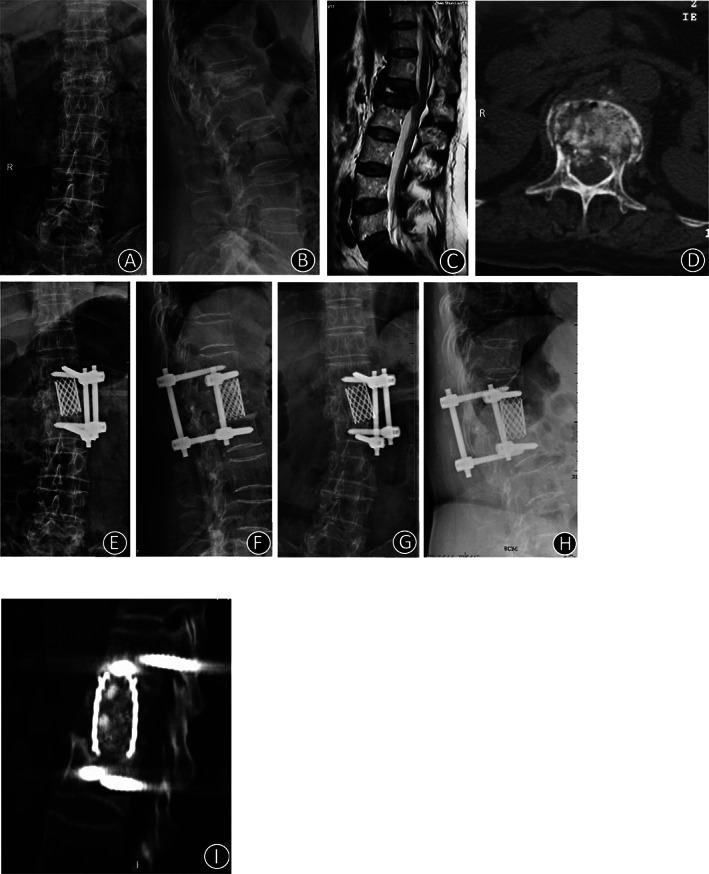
Clinical imaging from one representative patient (71‐year‐old woman) who complained of lumbar 1 compression fracture. (A, B) X‐ray images of lumbar vertebrae anteroposterior and lateral position X‐ray films showed L1 vertebral compression fracture. (C) T2‐weighted sagittal preoperative MRI of the lumbar spine showed that the spinal cord was compressed by the bone fragment. (D) The lumbar vertebrae CT indicates that the bone fragment had burst into vertebral canal. (E, F) At 3 days postoperatively, the X‐ray imaging indicated that the location of the internal fixator was excellent. (G,H) At 12 months postoperatively, the X‐ray imaging indicated that the location of the internal fixator was excellent. (I) At 12 months postoperatively, two‐dimensional CT indicates that the auto graft bone fusion was excellent.
